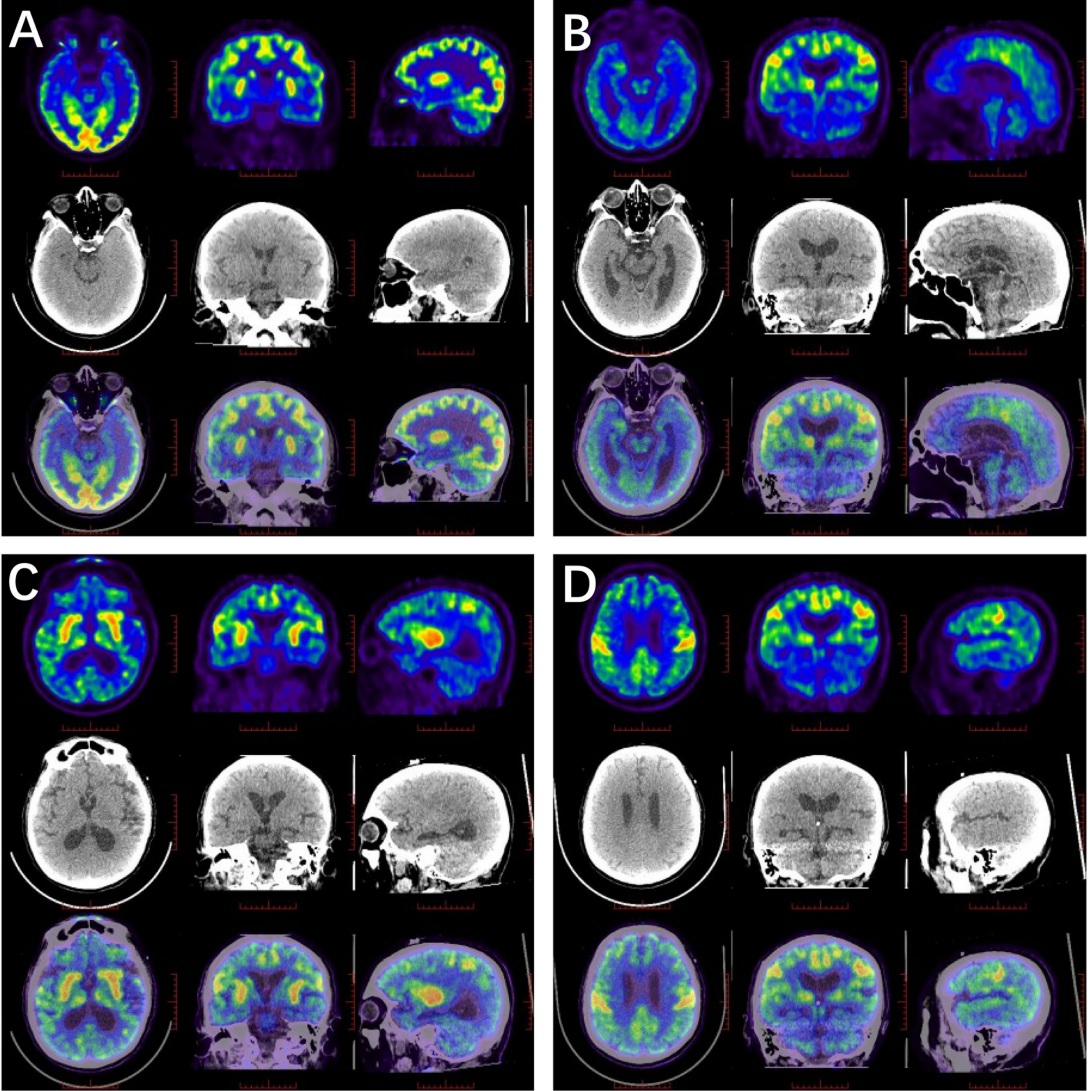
^18^F-FDG PET/CT scan in anti-amphiphysin encephalitis. (A) Glucose metabolism decreased in the right hippocampus (Patient #1). (B–D) High FDG uptake in the bilateral central cortex, left fronto-parietal para-sagittal, bilateral caudate heads, putamen and right thalamus, while the remaining brain cortex was diffusely and slightly decreased (Patient #4).
